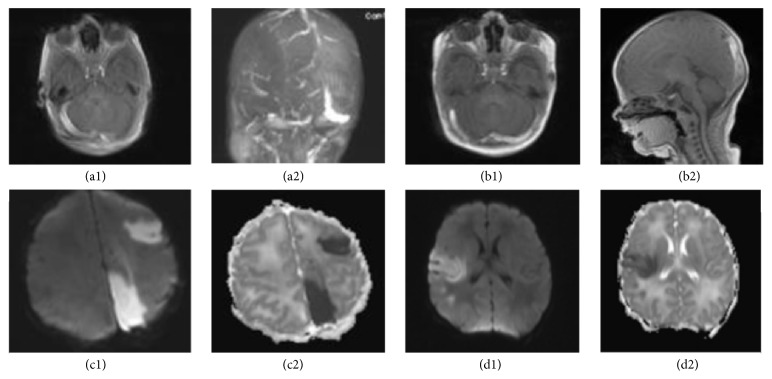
(a1, a2) Preterm neonate with severe perinatal depression born to mother with no prenatal care: the MRI and MRV images, respectively, show venous sinus thrombosis involving the entire right transverse and sigmoid sinus, most of the straight sinus, and the medial aspect of the left transverse sinus. In this case low molecular weight heparin was initiated. (b1, b2) Term neonate with sepsis, hyperthermia, and suspected episodes of seizures. On T1 images there was an abnormal signal intensity in the lower superior sagittal sinuses as well as bilateral transverse sinuses, consistent with CSVT. A small amount of intraventricular hemorrhage in the posterior body and atrium of the right lateral ventricle was also identified. Infant's coagulation work-up was negative for any major clotting disorder. (c1, c2) Diffusion weighted images (DWI) as well as ADC Map are shown for a term neonate with no identified risk factors that presented with seizures. (d1, d2) An IUGR infant admitted to the NICU due to suspected apnea and respiratory distress. An MRI without contrast demonstrated a moderately sized acute cortical infarct in the right middle cerebral artery territory, involving the right posterior frontal lobe and frontoparietal junction at the region of the motor strip.
